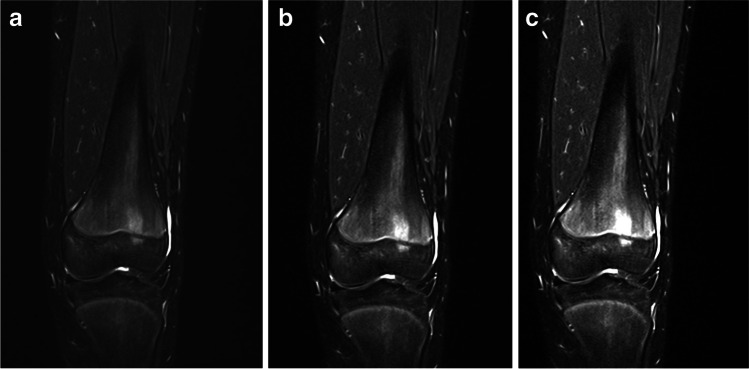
a–c MRI, coronal T2-W Dixon water-only of the knee in a healthy and asymptomatic 14-year-old girl. The perceived intensity level of the periphyseal bone marrow hyperintensity in the distal femur varies considerably with different window (*W*) and level (*C*) settings: (a) C192/W501, (b) C122/W271 and (c) C96/W198
